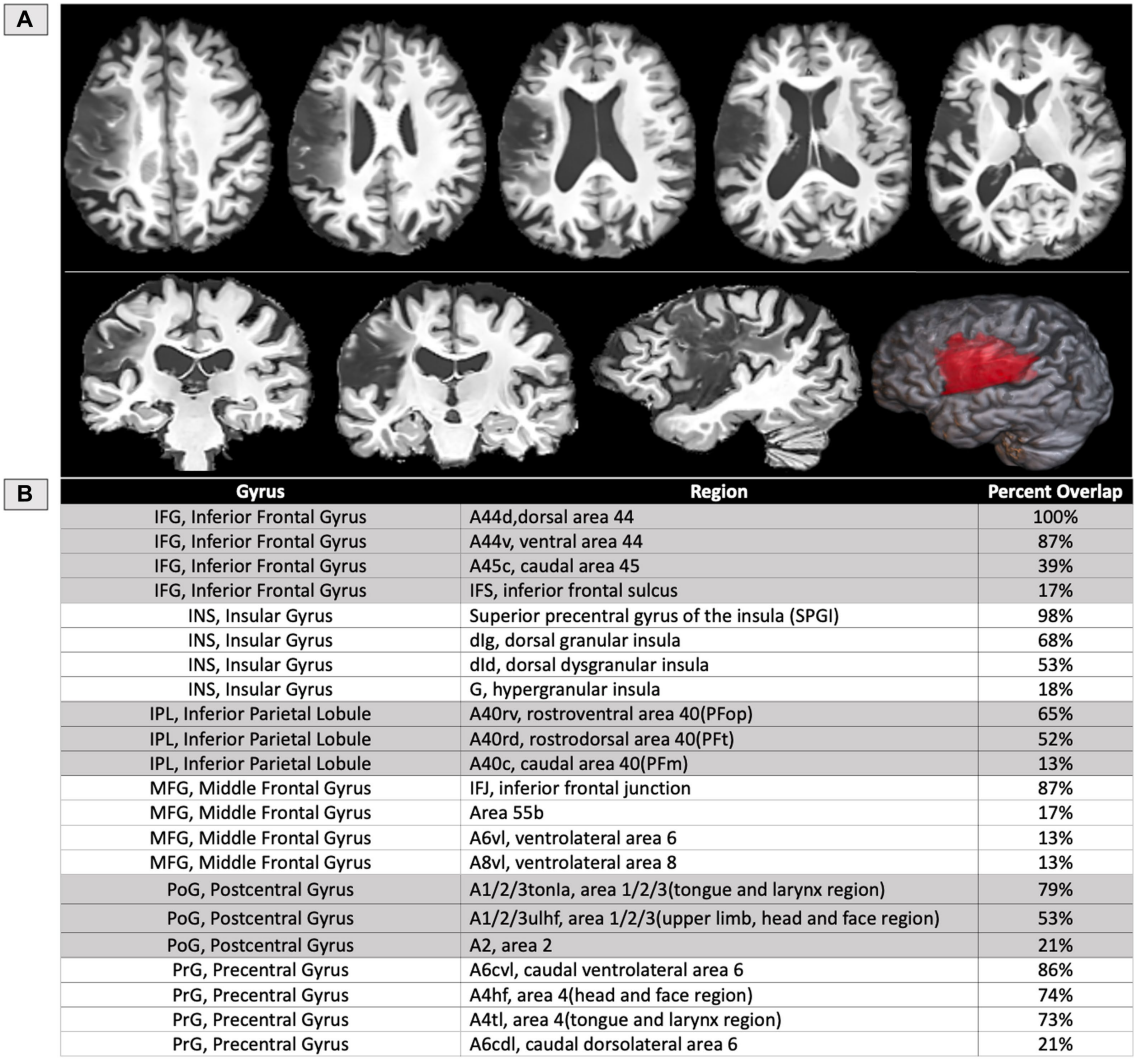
(A) T1 structural MRI of the participant’s lesion at 12 months post-onset shown on axial, sagittal, and coronal slices, as well as rendered in 3D. (B) Table of the areas damaged at a threshold of 5% or more based on regions of interest from the Brainnetome atlas (39), except for the SPGI, which was constructed based on prior literature (13), and area 55b (22).
